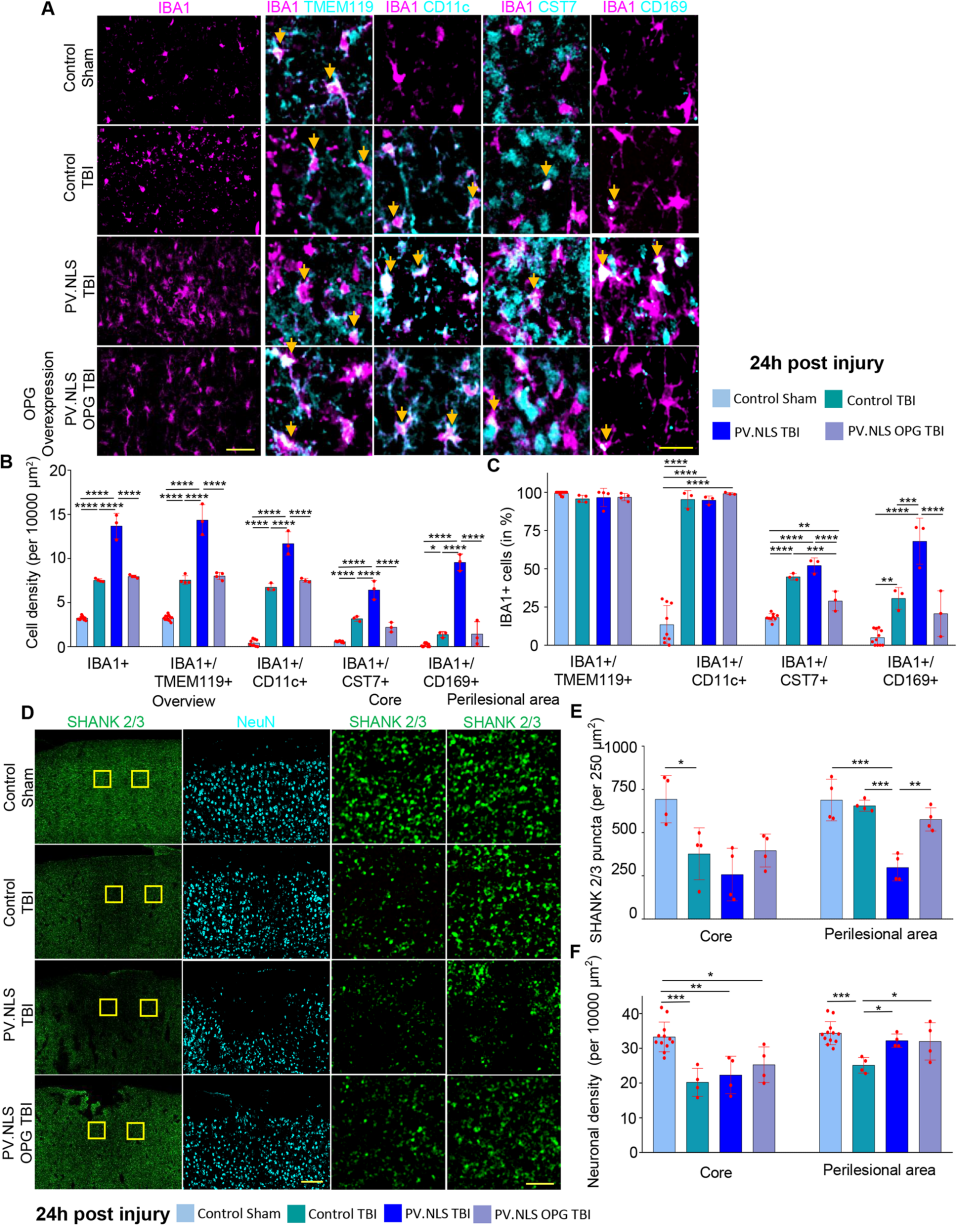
Re-expression of OPG in neurons with nuclear calcium buffering reduces microgliosis and prevents synaptic degradation after TBI. A–C Buffering of nuclear calcium signaling significantly increased the density of IBA1 + /TMEM119 + , IBA1 + /CD11c + , IBA1 + /CST7 + and IBA1 + /CD169 + cells 24 h after TBI compared to TBI alone (CT vs PT; for IBA1 + /TMEM119 + 7.56 ± 0.48 vs 14.37 ± 1.74; for IBA1 + /CD11c + 6.74 ± 0.42 vs 11.70 ± 1.33; for IBA1 + /CST7 + 3.17 ± 0.29 vs 6.44 ± 1.05; for IBA1 + /CD169 + 1.38 ± 0.329 vs 9.56 ± 0.934). Re-expression of OPG decreased IBA1 + /TMEM119 + , IBA1 + /CD11c + , IBA1 + /CST7 + ; IBA1 + /CD169 cells compared to PV.NLS TBI (PT vs POT; for IBA1 + /TMEM119 + cells 14.37 ± 1.74 vs 8.02 ± 0.40; for IBA1 + /CD11c + cells 11.7 ± 1.33 vs 7.52 ± 0.21; for IBA1 + /CST7 + cells 6.44 ± 1.05 vs 2.20 ± 0.54; for IBA1 + /CD169 + 9.56 ± 0.93 vs 1.44 ± 1.38). Likewise, re-expression of OPG normalized the fraction of IBA1 + /CST7 + and IBA1 + /CD169 + compared to PV.NLS TBI (PT vs POT; for CST7 + 52.14 ± 4.91% vs 28.98 ± 6.44%; for CD169 + 68.01 ± 15.06% vs 20.67 ± 14.95%). *N* = 3. Mean ± SD Scale bar: 40 µm (overview) and 20 µm (insert). D, E Re-expression of OPG together with buffering of nuclear calcium signaling did not alter core synaptic density compared to PV.NLS-TBI but significantly increased the synaptic density in the perilesional area compared to PV.NLS-TBI (PT vs POT; 298.3 ± 78.1 vs 576.3 ± 66.8). *N* = 4. F OPG re-expression together with nuclear calcium buffering did not alter core neuronal density compared to TBI alone but significantly increased neuronal density in the perilesional areas (CT vs POT 25.13 ± 2.26 vs 32.01 ± 5.38). Yellow inserts show analyzed core and perilesional areas. Scale bar: 100 µm (overview) and 5 µm (insert). Data are shown as mean ± SD. *N* = 4. p* < 0.05; *p* < 0.01; p* < 0.001
